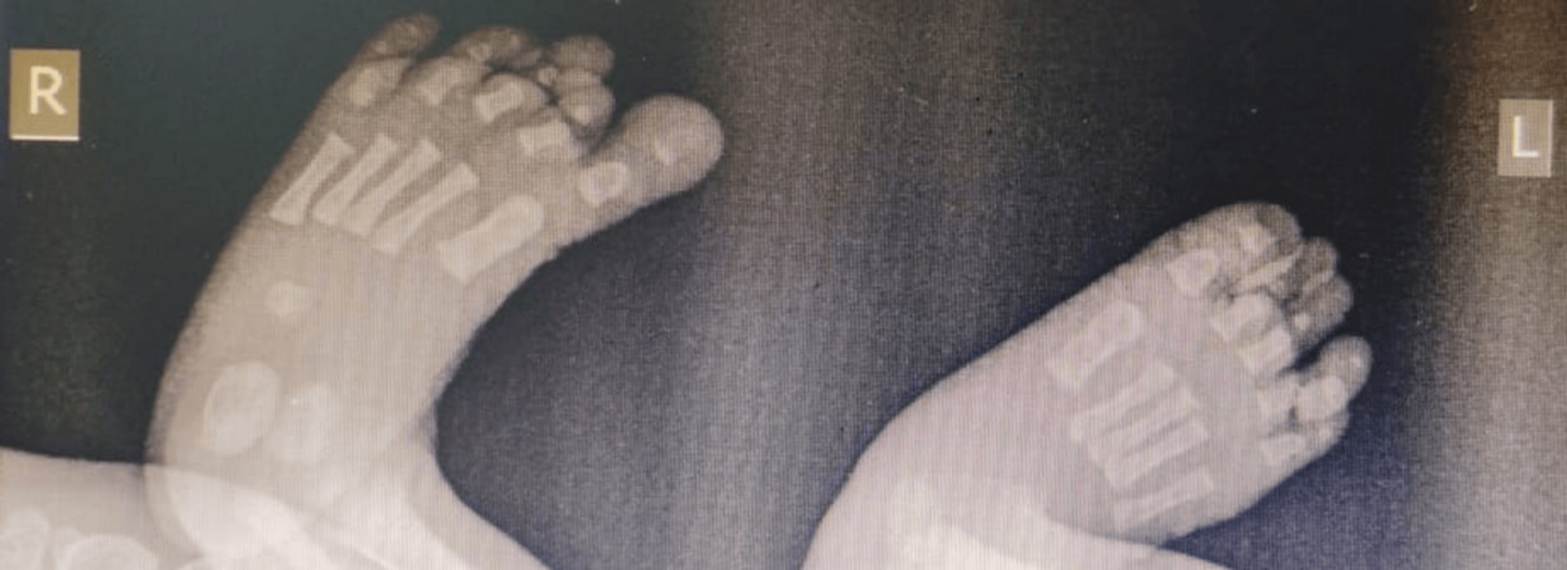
Radiograph of the feet showing the presence of all distal phalanges confirming the absence of associated bony defects
